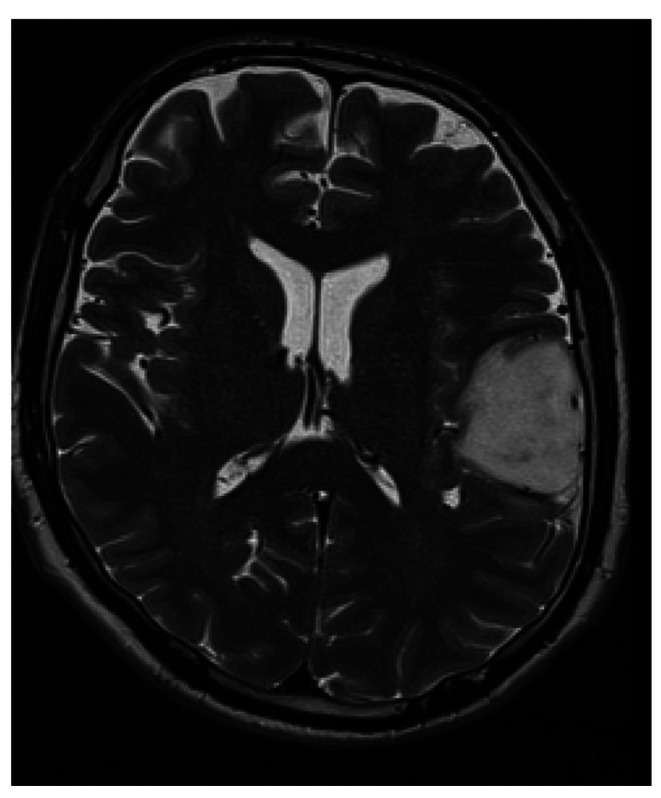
LGG before volumetric segmentation in T2 weighted MRI.
